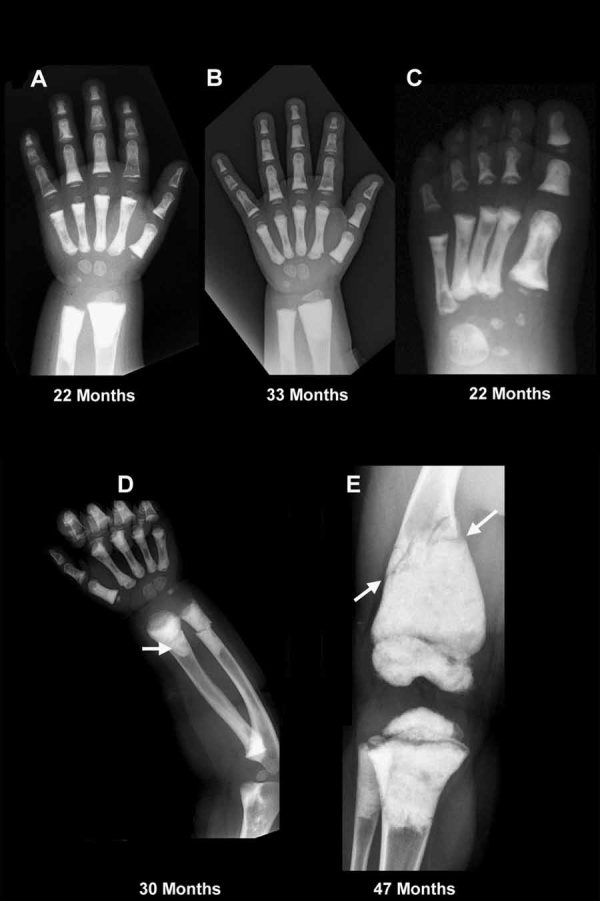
Hand and foot radiographs. At 22 (*A*) and 33 (*B*) months of age the left hand and at 22 (*C*) months of age the left foot show diffuse sclerosis greatest in the metaphyses of the metacarpals and metatarsals and distal radius and ulna. The amount of sclerosis in the proximal phalanges varies from much to little and is greatest in the metaphyseal regions. The tarsals have some sclerosis greatest in the cuboids. Pathologic fractures are shown through sclerotic bone in the distal radius (*arrow*) and ulna in the anteroposterior projection (*D*) at 30 months and near nonsclerotic bone in the right femur (*arrows*) at 47 months (*E*).
